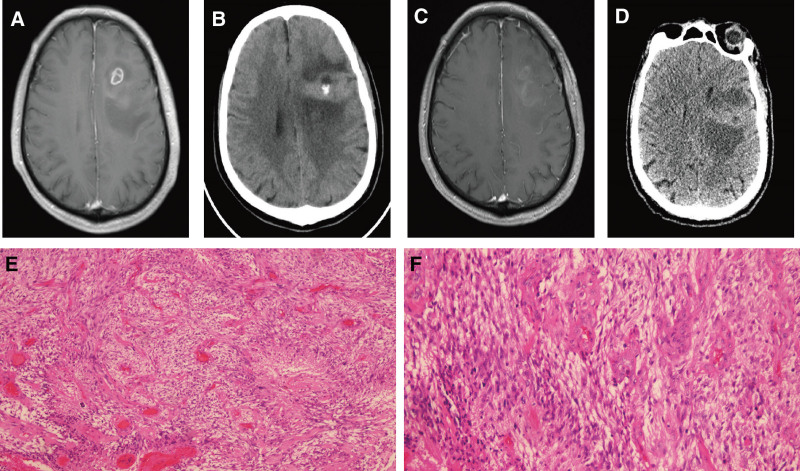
Clinical data of patient number 4. (A) preoperative MRI shows a cystic solid enhancing lesion in the frontal lobe. (B) CT shows a nodular high density lesion in the left frontal lobe. (C, D) Postoperative images show that the majority of the tumor was resected. (E, F) Diffuse glioma characterized by abundant tumor cells, marked cellular atypia, visible mitotic figures, palisading necrosis, and glomeruloid microvascular proliferation. Tumor cells exhibited diffuse growth with high cellular density (left, ×100). Focal areas showed marked cellular atypia, accompanied by necrosis and vascular proliferation (right, ×200). CT = computerized tomography, MRI = magnetic resonance imaging.
